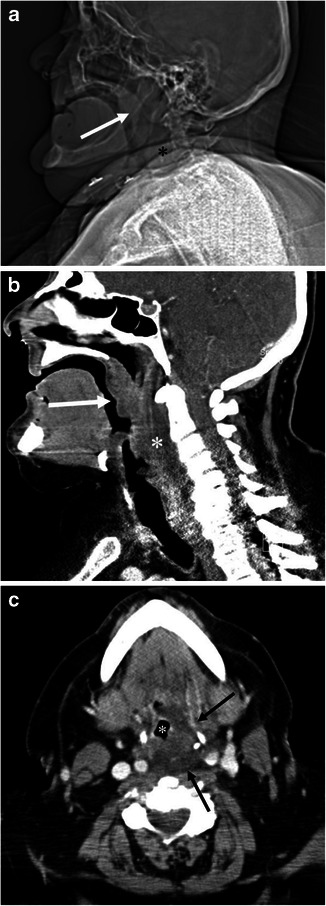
A 68-year-old male with angiotensin converting enzyme (ACE) inhibitor-induced angioedema involving the soft palate, pharynx and larynx. a Scout view of the neck computed tomography (CT) shows enlargement of the soft palate (*arrow*) and thickening of the retropharyngeal soft tissue (*asterisk*). b The sagittal reformatted contrast enhanced (CE)-CT shows marked swelling of the soft palate (*arrow*) and posterior pharyngeal wall (*asterisk*) with decreased attenuation. c The axial CE-CT image shows swelling of the left posterolateral retropharyngeal space (*arrows*), and narrow displaced pharyngeal airway (*asterisk*)
